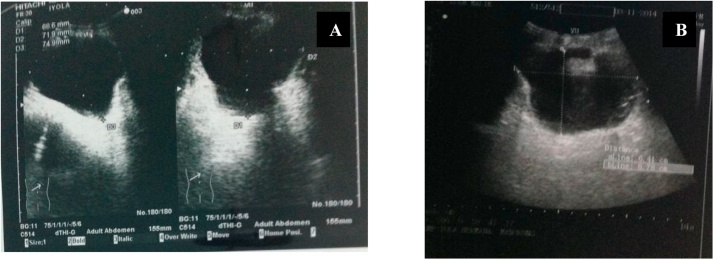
The patient’s post-bladder augmentation ultrasound (A. 1 month after surgery, B. 2 months after surgery.
